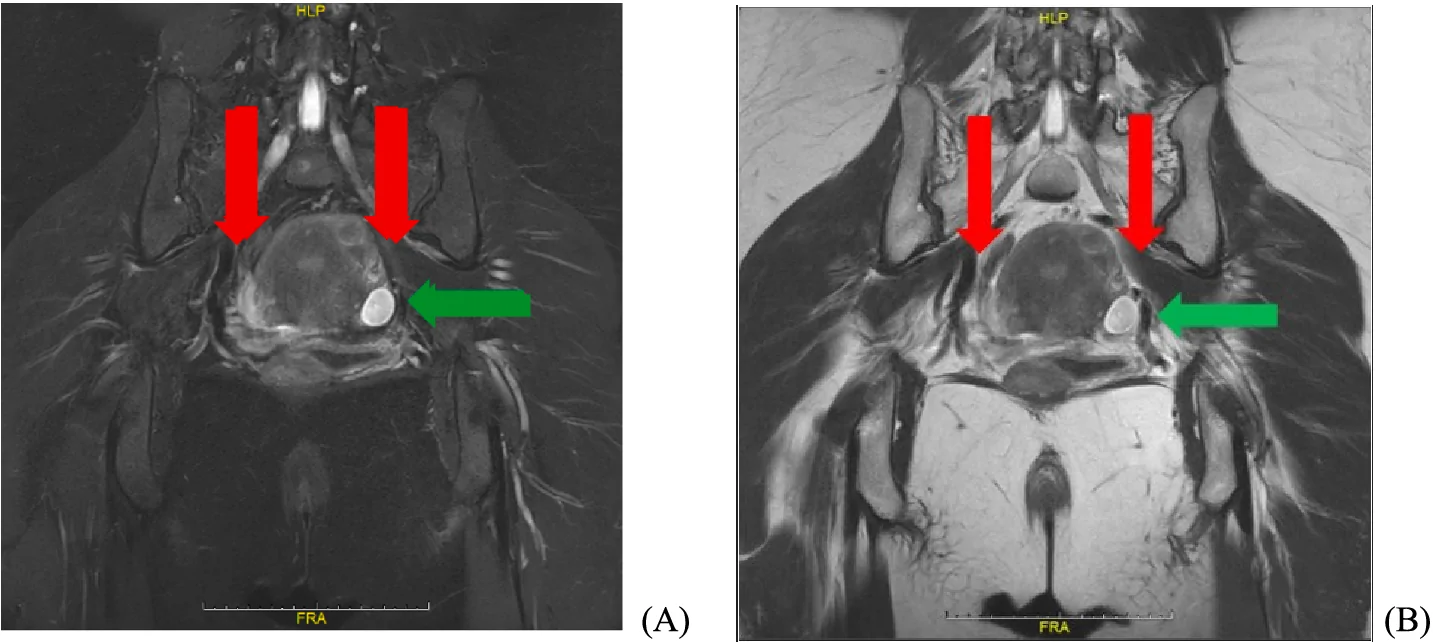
Coronal fat-suppressed T2-weighted (A) and T2-weighted (B) magnetic resonance image of the pelvis. The image clearly delineates a well-circumscribed, round, highly hyperintense cystic lesion measuring approximately 1.26 × 1.14 cm in the left adnexal region (green arrow). Both ovaries are poorly visualized (red arrow), making detailed assessment of antral follicles (AFs) impossible.
